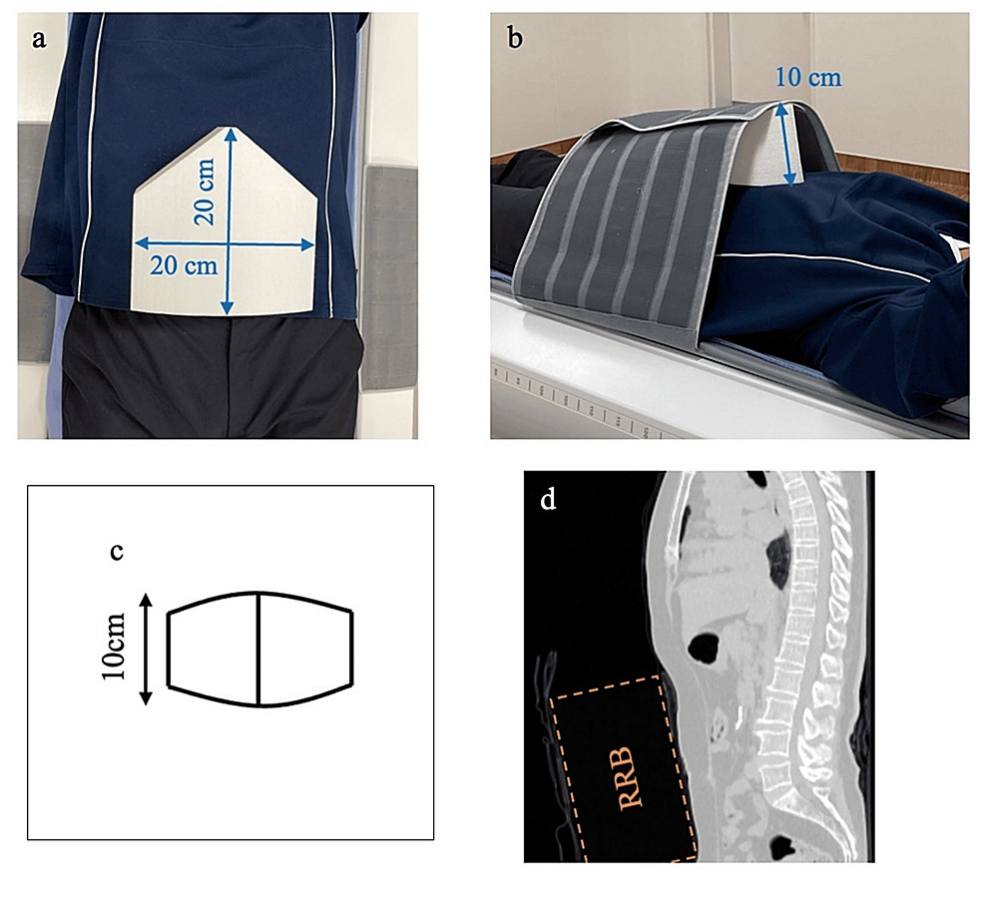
The respiratory motion reduction block (a) A pentagonal styrene foam device with a width of 20 cm and a length of 20 cm. (b) The RRB was tightly wrapped using the belt of the bed. (c) View of the RRB from the apex. (d) Computed tomography sagittal image of a female of Japanese standard proportions above 70 years old. RRB: Respiratory motion reduction block.
